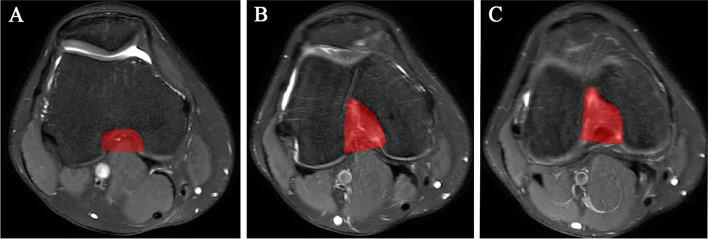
Axial slices of knee MRI showing the measurement of femoral intercondylar notch volume. A The most proximal level of the intercondylar notch. B One of the middle levels of the intercondylar notch. C The most distal level of the intercondylar notch
